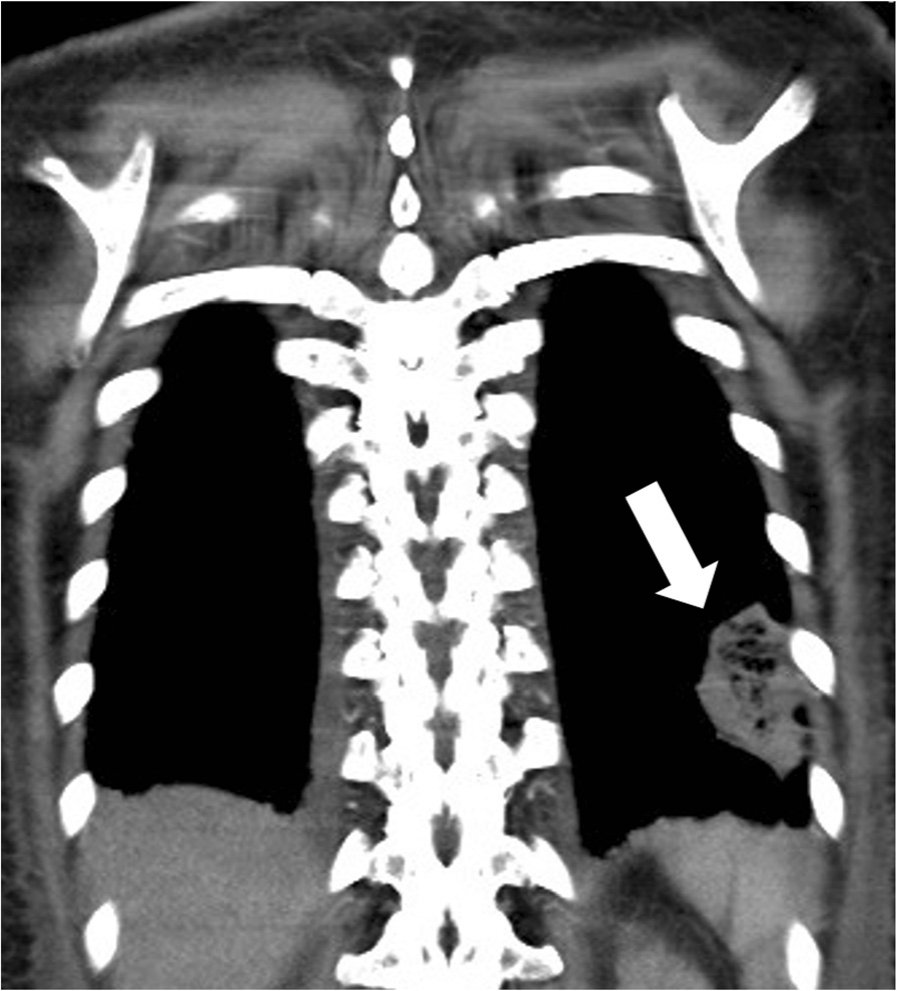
On enhanced chest computed tomography (CT), a consolidated patch with central necrosis over the lower left lung (*white arrow*)
